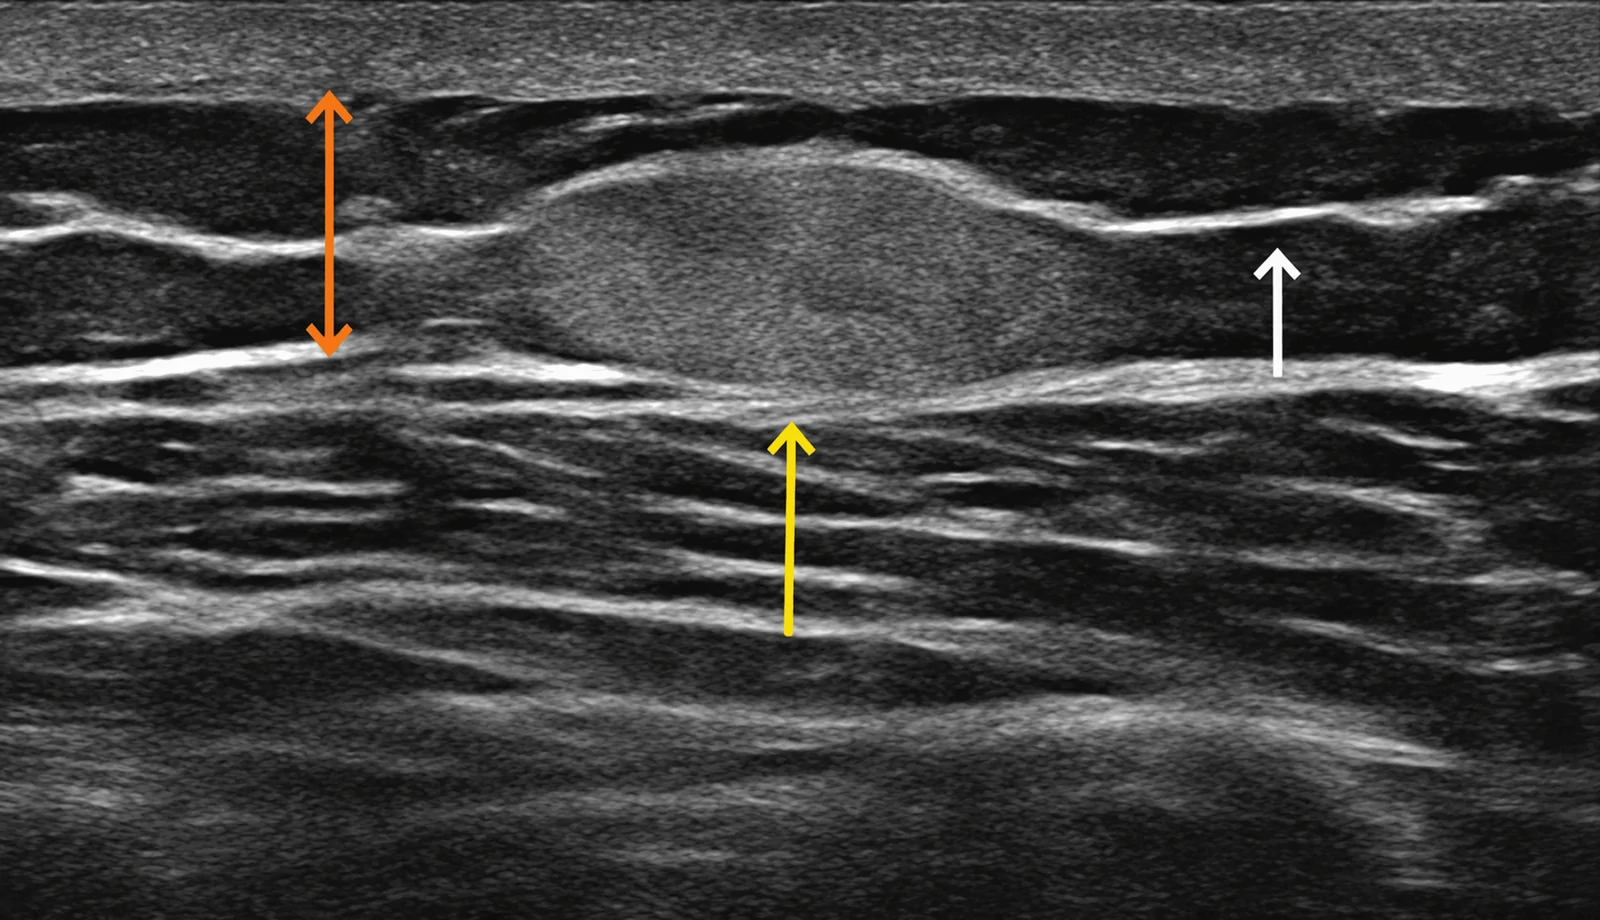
Ultrasound of a thigh angiolipoma Ultrasonographic image of the thigh in a patient with angiolipoma demonstrating normal hypodermis thickness (orange arrow) and an oval hyperechoic nodule (yellow arrow) with regular margins, permeated by adipose tissue with preserved architecture (intact linear echogenic septa, white arrow) and a regular dermal-hypodermal junction.
